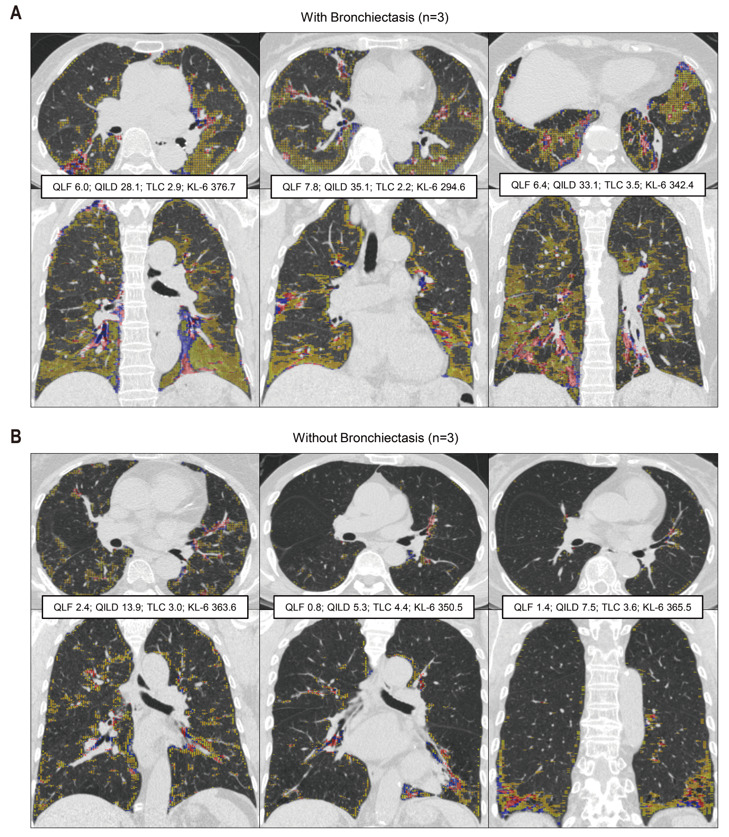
Two distinct patterns of association between QILD score and serum KL-6 level were presented by representative cases having similar serum KL-6 levels (cohort 2). (A) HRCT scans of 3 cases with bronchiectasis; QLF 6.7 ± 0.9, QILD 32.1 ± 3.6, TLC 2.9 ± 0.5 L, and KL-6 337.9 ± 41.2 U/mL. (B) HRCT scans of 3 cases without bronchiectasis; QLF 1.5 ± 0.8, QILD 8.9 ± 4.5, TLC 3.6 ± 0.6 L, and KL-6 359.9 ± 8.2 U/mL. Mean ± standard deviation; Upper row: transverse view; Lower row: coronal view; QLF = sum of red and blue dot, QGG = yellow dot.
